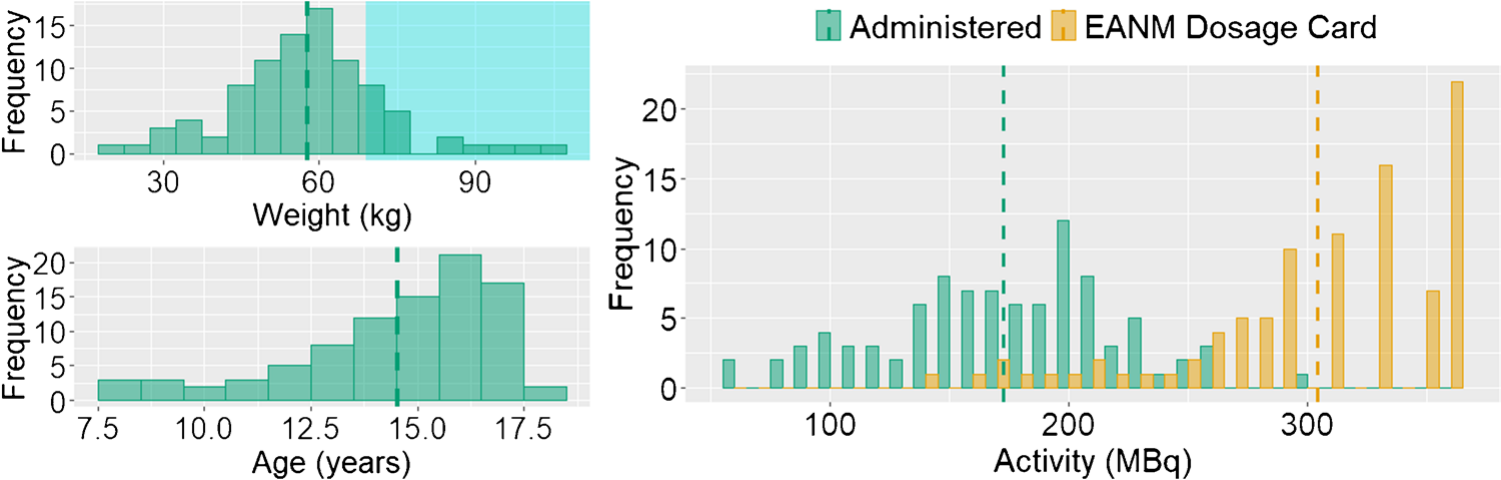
Baseline characteristics of the subset of 91 selected [^18^F]FDG scans from the EuroNet-PHL-C2 trial. Histograms of weight (top left, bin size = 5 kg), age (bottom left, bin size = 1 year) and administered activity as well as activity recommended by the EANM Paediatric Dosage Card (right, bin size = 10 MBq). Vertical dashed lines indicate the mean activities. The cyan box marks the weight range > 68 kg not defined by the EANM Paediatric Dosage Card
